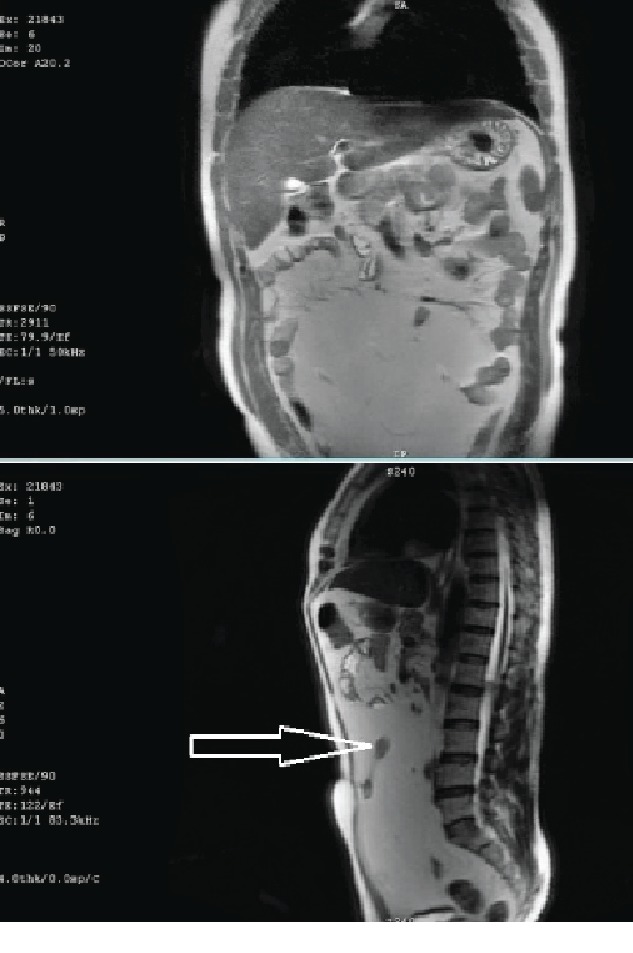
Abdominal and pelvic MRI detecting mesenteric lipomatous tumor encroaching the intestinal loop (white arrow).
